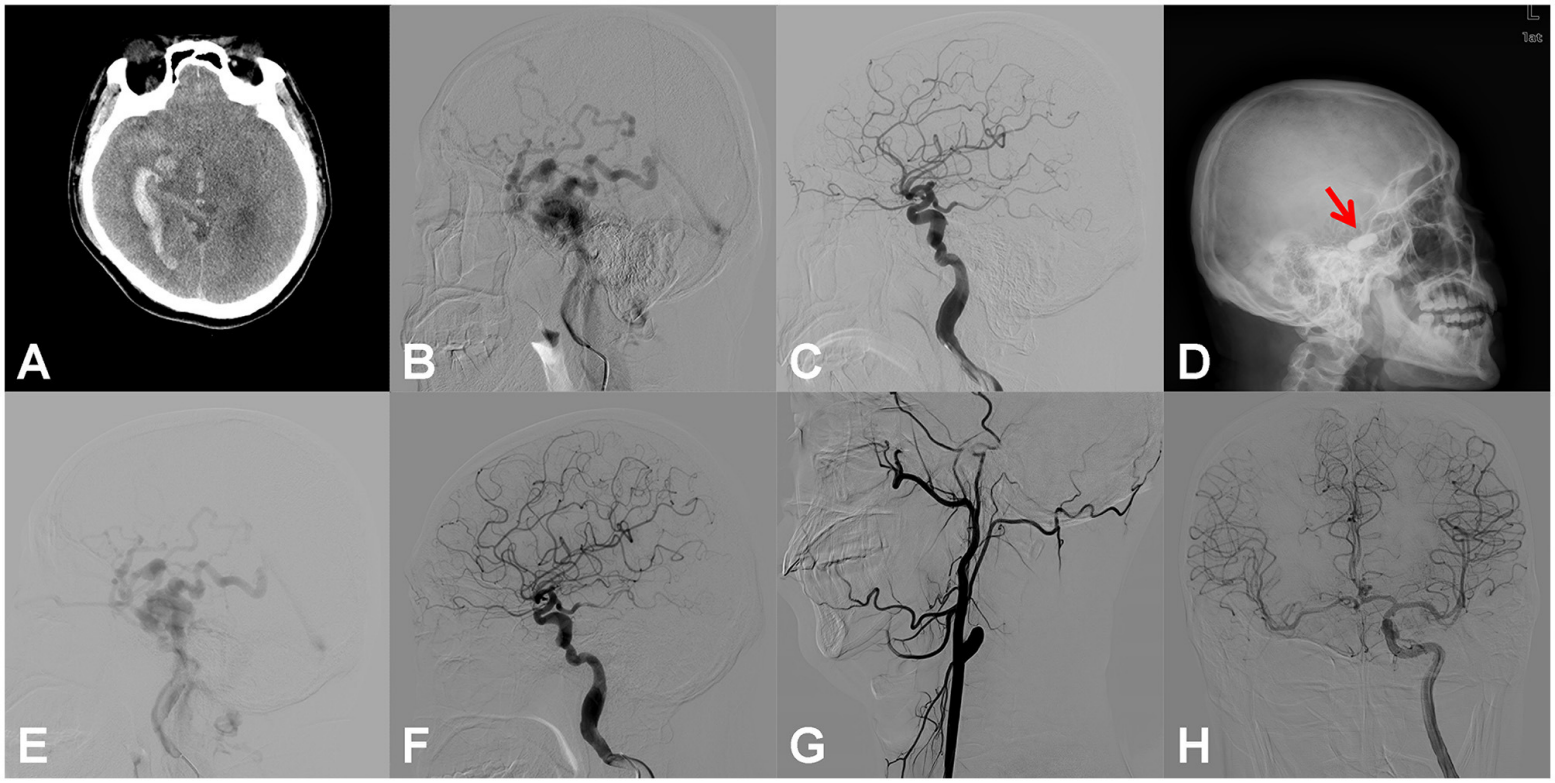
Right carotid cavernous fistula (CCF). An adult patient presented to the emergency room with an onset of severe headache for 6 h. Right temporal auscultation had a blowing murmur that was consistent with a heartbeat. (A) Emergency head computed tomography (CT) showed subarachnoid hemorrhage, right temporal lobe, and ventricular hematoma. (B) Digital subtraction angiography (DSA) of the right internal carotid artery (ICA) showed the CCF, with reflux drainage of cortical veins. (C) After the dilation of two detachable balloons (Goldbal2), the pathological shunt disappeared, and the cerebral blood flow recovered. (D) The patient suffered from sudden throbbing tinnitus on the left side 2 days after the endovascular procedure, and the head X-ray radiography revealed that only one balloon developed (red arrow). (E) Emergency DSA showed the recurrence of CCF. (F) With the introduction of another detachable balloon (Goldbal2) in the cavernous sinus, the pathological shunt disappeared angiographically and the patient recovered well without any neurological deficits. (G) DSA follow-up 6 months after the procedure showed complete occlusion of the lesional ICA. (H) DSA of the left ICA showed that the perfusion of the right hemisphere was compensated adequately by the anterior communicating artery.
